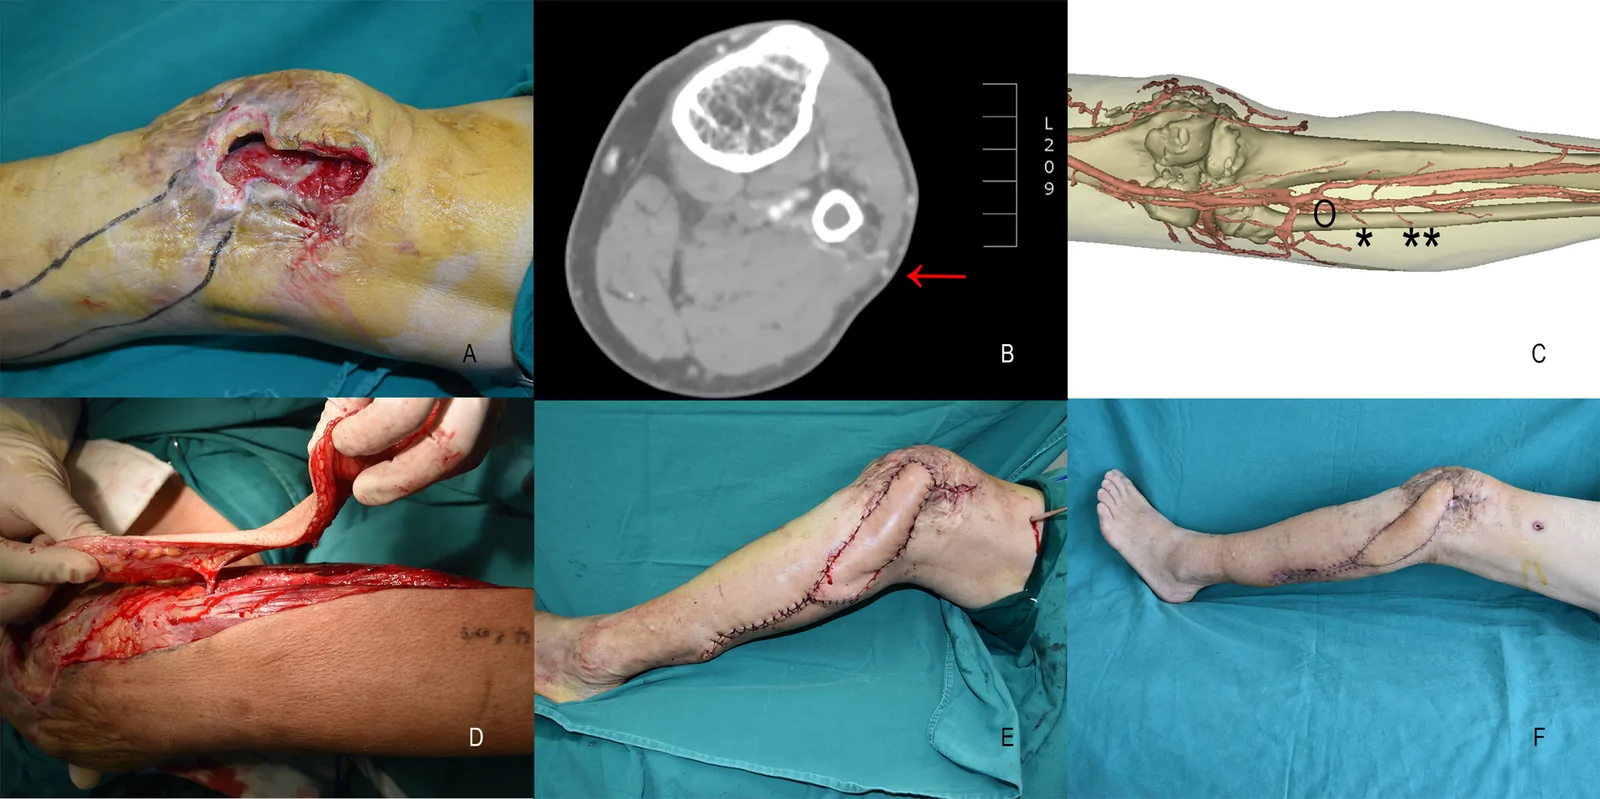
(A) Preoperative view showing a 7 × 4 cm soft tissue defect on the lateral aspect of the left knee. B Preoperative CTA image showing the location of perforator 1, indicated by the red arrow. C Mimics 20.0 reconstruction demonstrating two perforators arising from the peroneal artery. O, source vessel; *, perforator 1; , perforator 2. D Intraoperative view after harvest of a 25 × 4 cm peroneal artery perforator flap without muscle dissection. E Immediate postoperative appearance after flap inset. F One-month postoperative photograph
